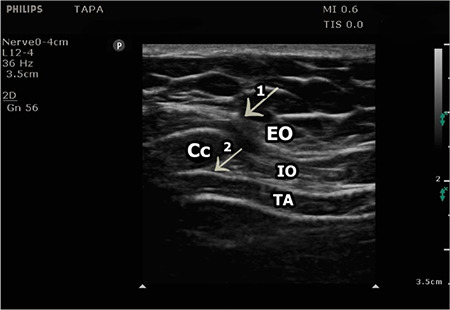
Sonographic anatomy of TAPA and M-TAPA. Arrows indicate the needle directions. 1 + 2 = TAPA, only 2 = M-TAPA. Cc, costal cartilage; EO, external oblique muscle; IO, internal oblique muscle; TA, transversus abdominis muscle, M-TAPA, modified-through perichondrial approach.
